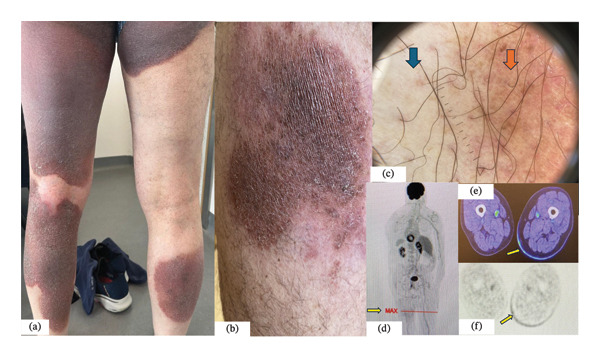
Clinical presentation and 18F‐FDG PET/CT imaging features. (a) Well‐circumscribed, reticulated, coalescent, nonpruritic dark red‐to‐brown patches involving the lower extremities. (b) Close‐up view of the poikilodermatous and hyperpigmented lesion of the anterior aspect of the right thigh. (c) Dermoscopic image revealing the typical features of poikiloderma blue arrow: normal skin, red arrow: poikilodermatous skin. (d) ^18^F‐FDG PET/CT imaging demonstrating focal hypermetabolic uptake within the thigh and lower extremity lesions (yellow arrow). Maximum intensity projection image showing hypermetabolic activity in the thigh. (e) Axial fused PET/CT image demonstrating focal subcutaneous hypermetabolic uptake in the thigh (yellow arrow). (f) Corresponding axial PET image confirming focal hypermetabolic activity (yellow arrow).
